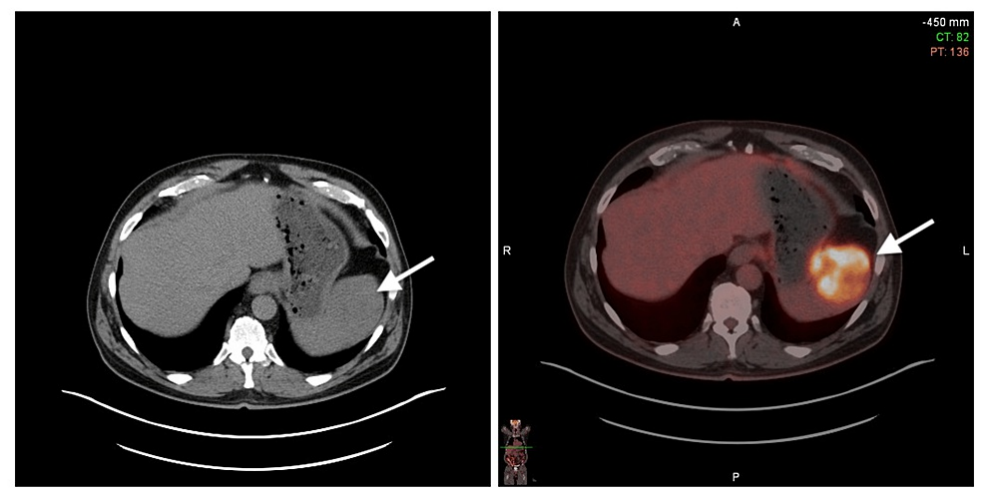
Transverse section of PET-CT scan at the level T10 showing FDG-avid splenic mass (arrow) PET-CT, positron emission tomography-computed tomography; FDG, fluorodeoxyglucose
